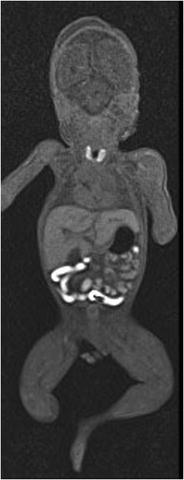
Coronal T1-weighted (W) image of a 24 weeks gestation fetus showing the normal T1 hypersignal of the meconium and thyroid. The fetal liver appears relatively hypersignal, probably due to high glycogen content
